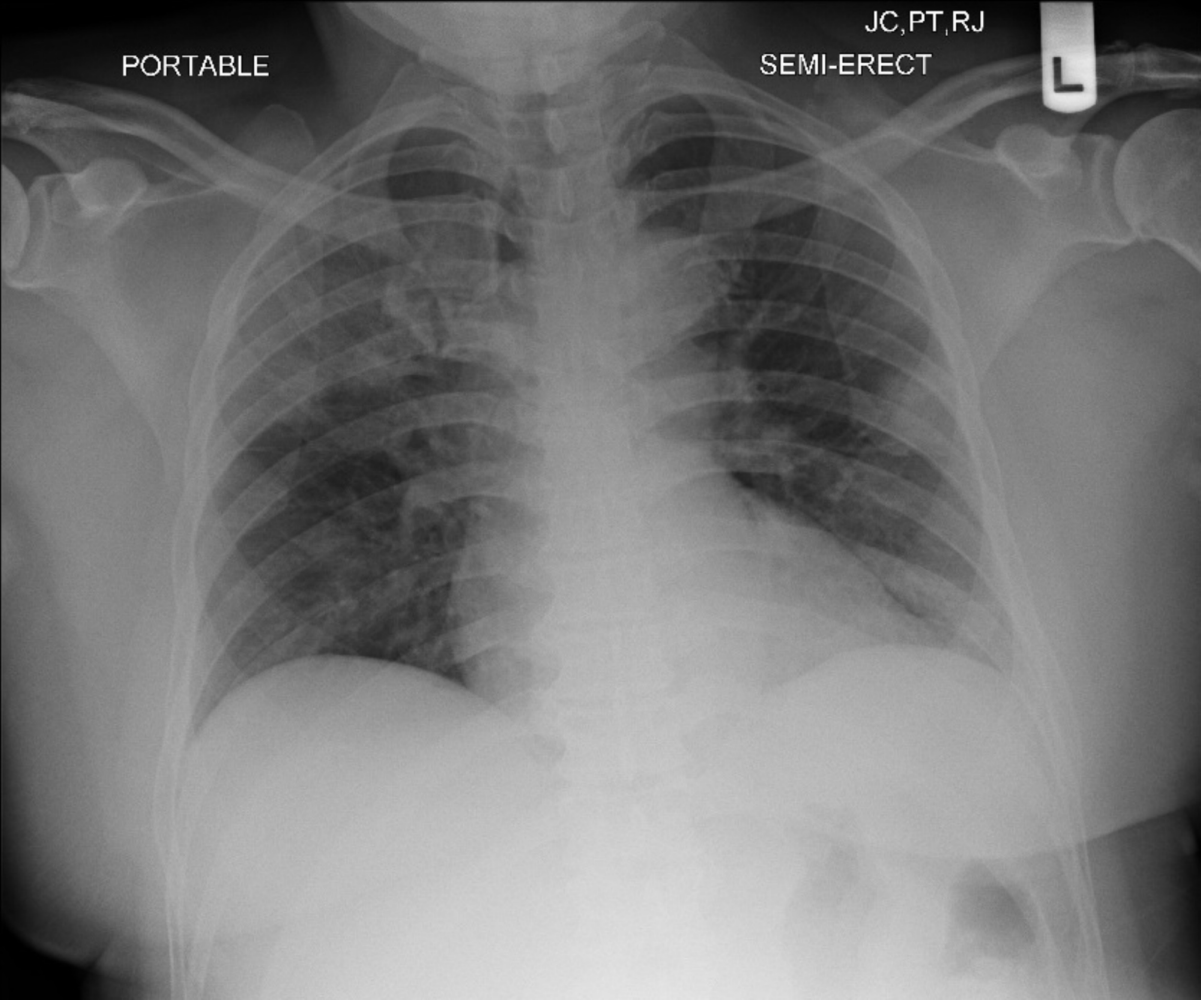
Chest X-ray (day 1) revealed right upper lobe and bilateral lower lobe infiltrates
